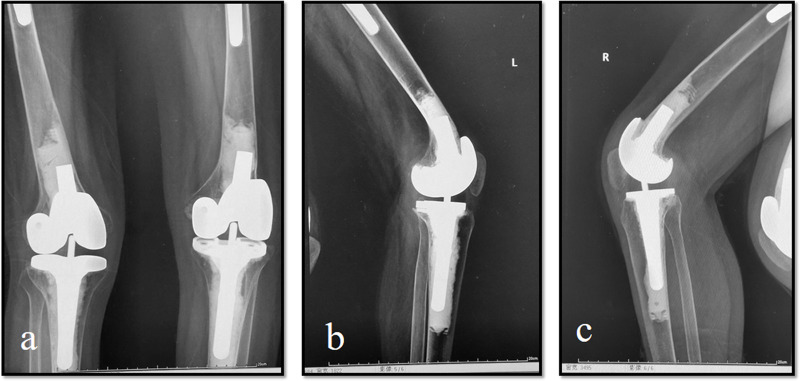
The range of motion and bony flexion contracture significantly improved after surgery. (a) Postoperative radiographs following bilateral total knee arthroplasty at 12 months. (b) Left knee can be flexed to 100° postoperatively. (c) Right knee can be flexed to 102° postoperatively.
